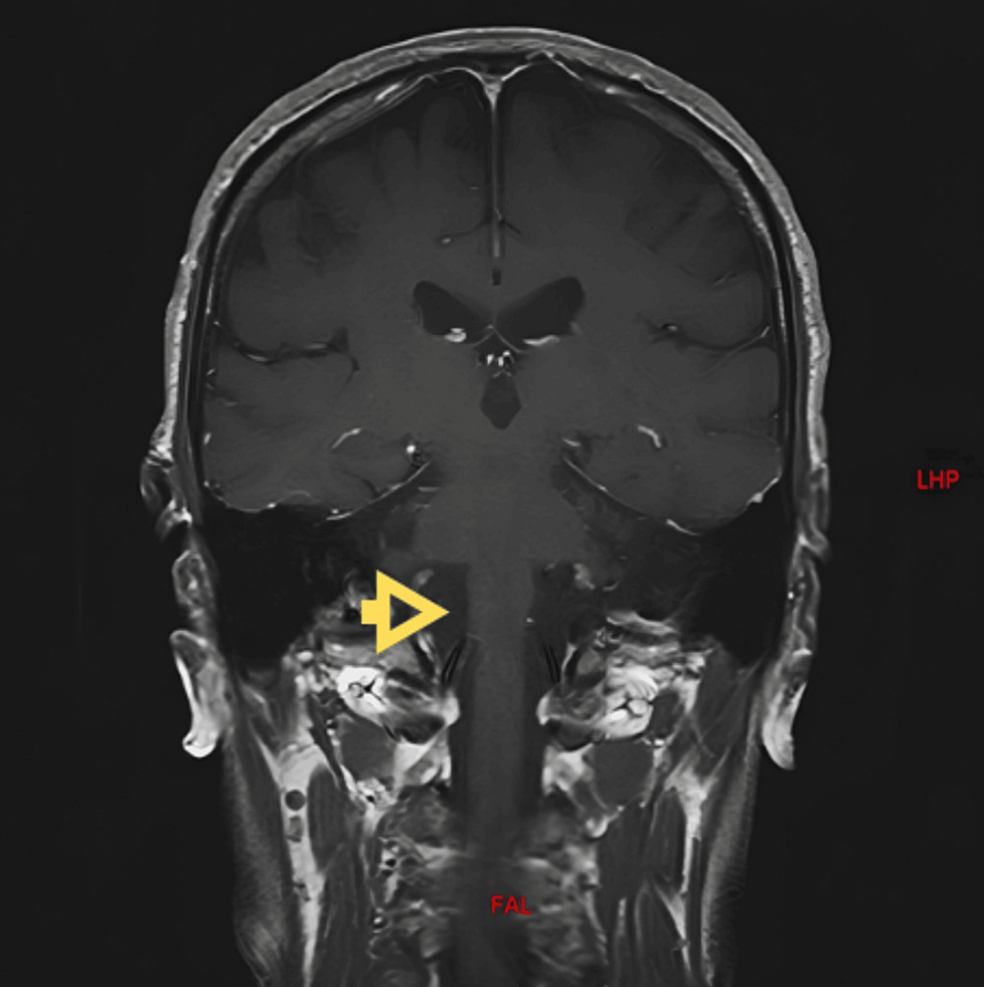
Brain MRI with gadolinium contrast enhancement in the coronal plane using a T1-weighted sequence In this image, significant bilateral cerebellar atrophy is observed, with preservation of the brainstem.
